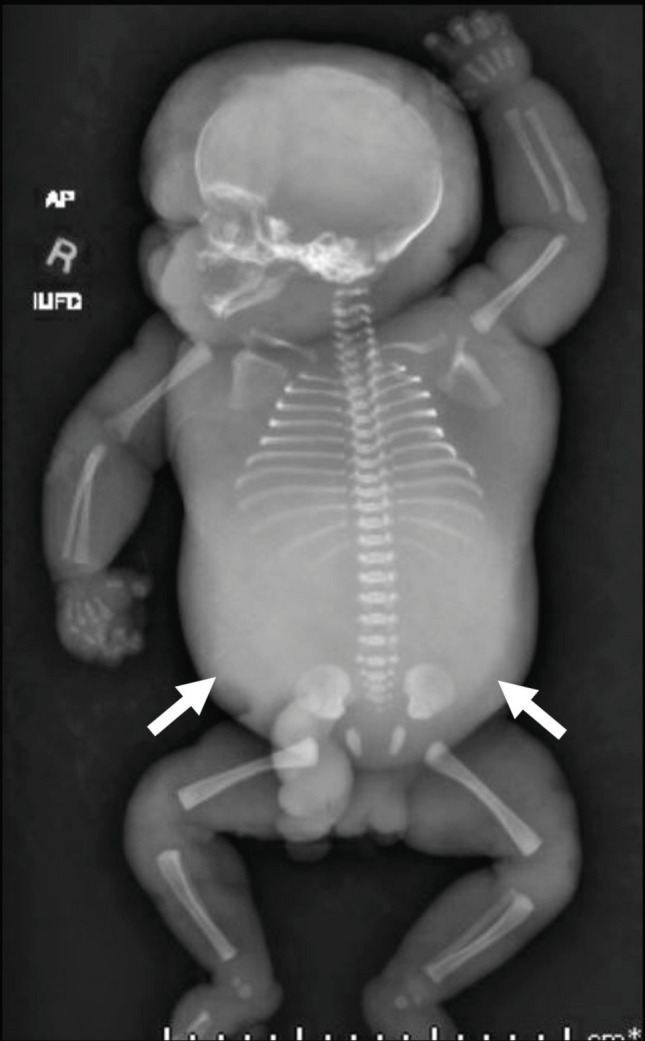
Postmortem radiograph of a 26-week gestational age fetus with congenital neuroblastoma and placental metastases. Anteroposterior radiograph (babygram) demonstrates generalized soft tissue edema and abdominal distension (*arrows*). No skeletal abnormalities are identified
